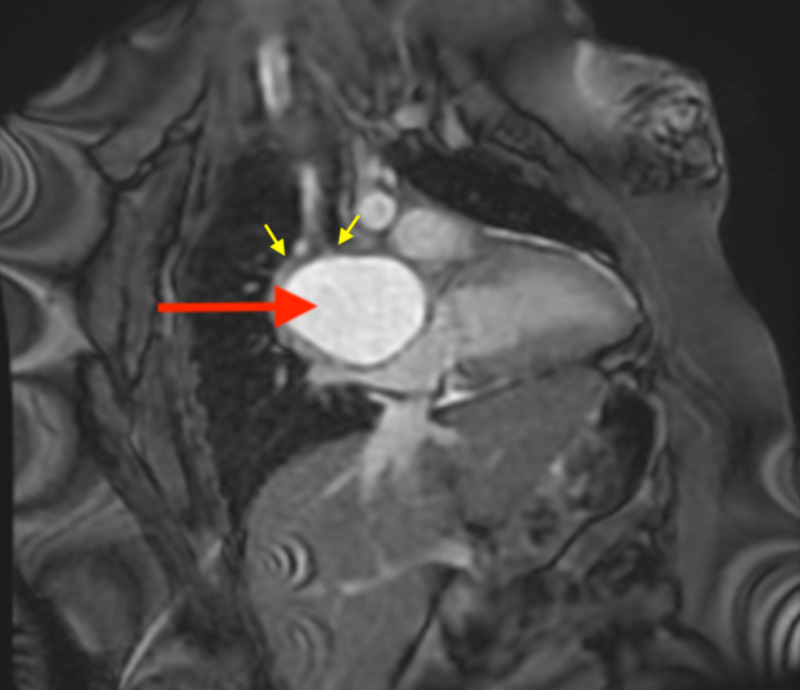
Cardiac MRI (FIESTA, fat-saturated two-chamber view) showing T2 hyperintense mediastinal mass (red arrow), splaying the mainstem bronchi (yellow arrows). FIESTA, fast imaging employing steady-state acquisition
